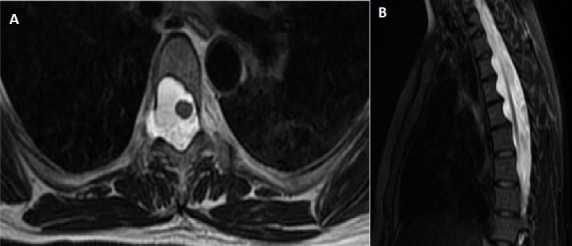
sagittal (A) and axial (B) T2-weighted spin-echo magnetic resonance image of the thoracic spine showing posterior vertebral scalloping by dural ectasia
